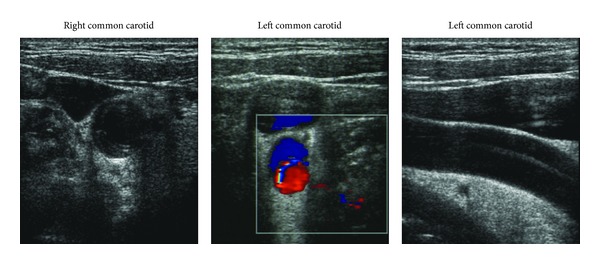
Doppler ultrasounds showing bilateral common carotid artery dissection.
